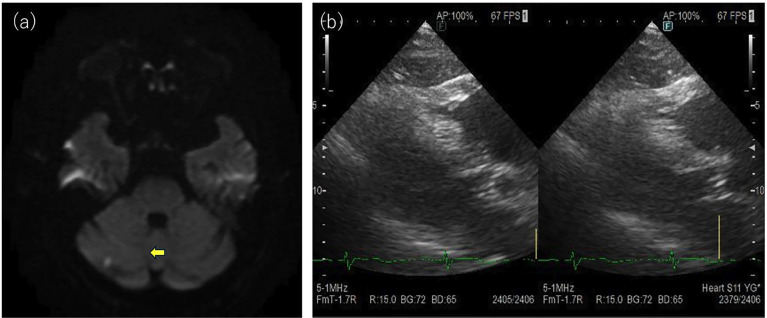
(a) Head magnetic resonance imaging revealed a microinfarction in the right cerebellum. (b) Echocardiography revealed no obvious vegetation.
